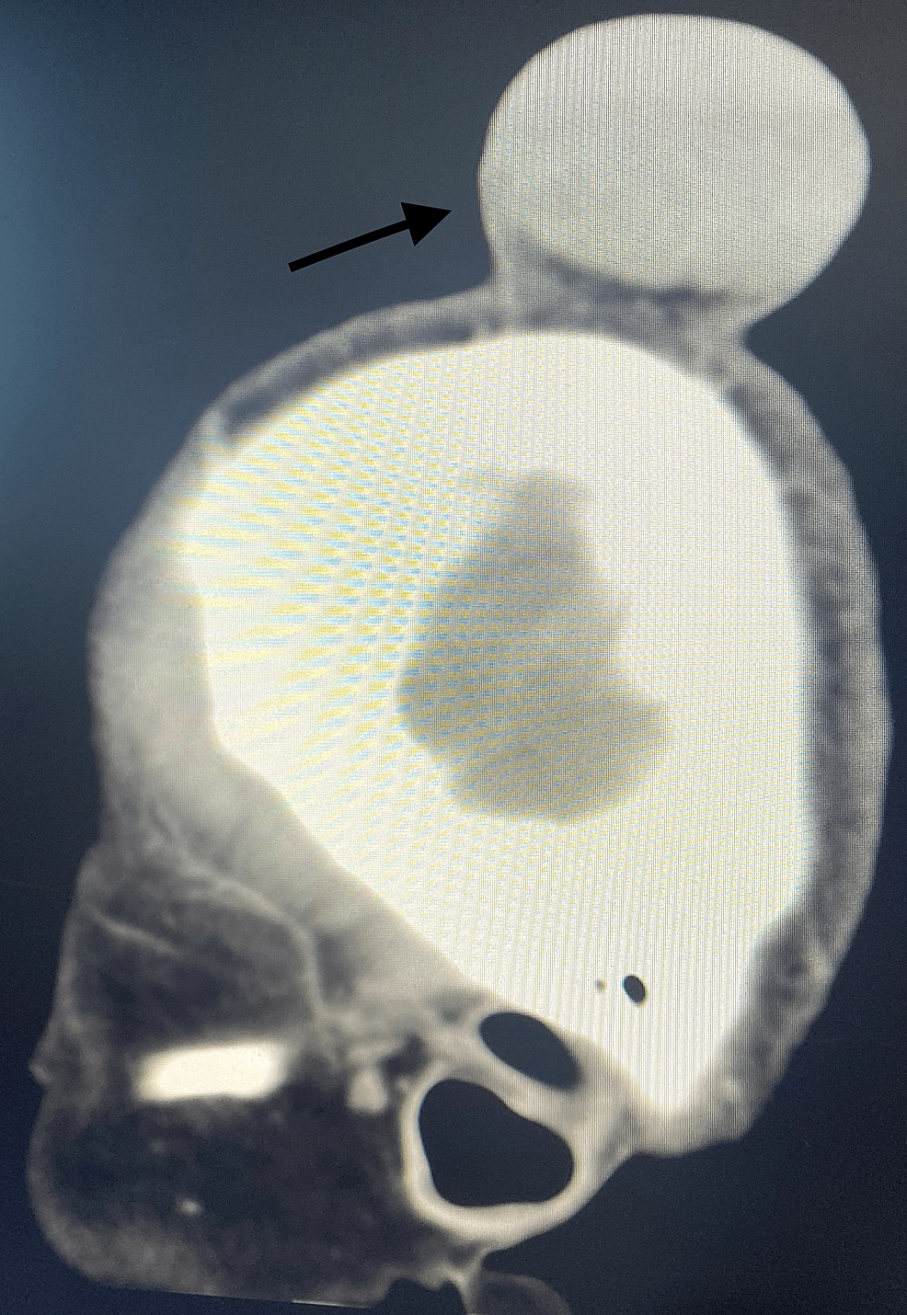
CT scan of the brain without contrast (sagittal view) showing significant sized right scalp swelling with no evidence of bony destruction
